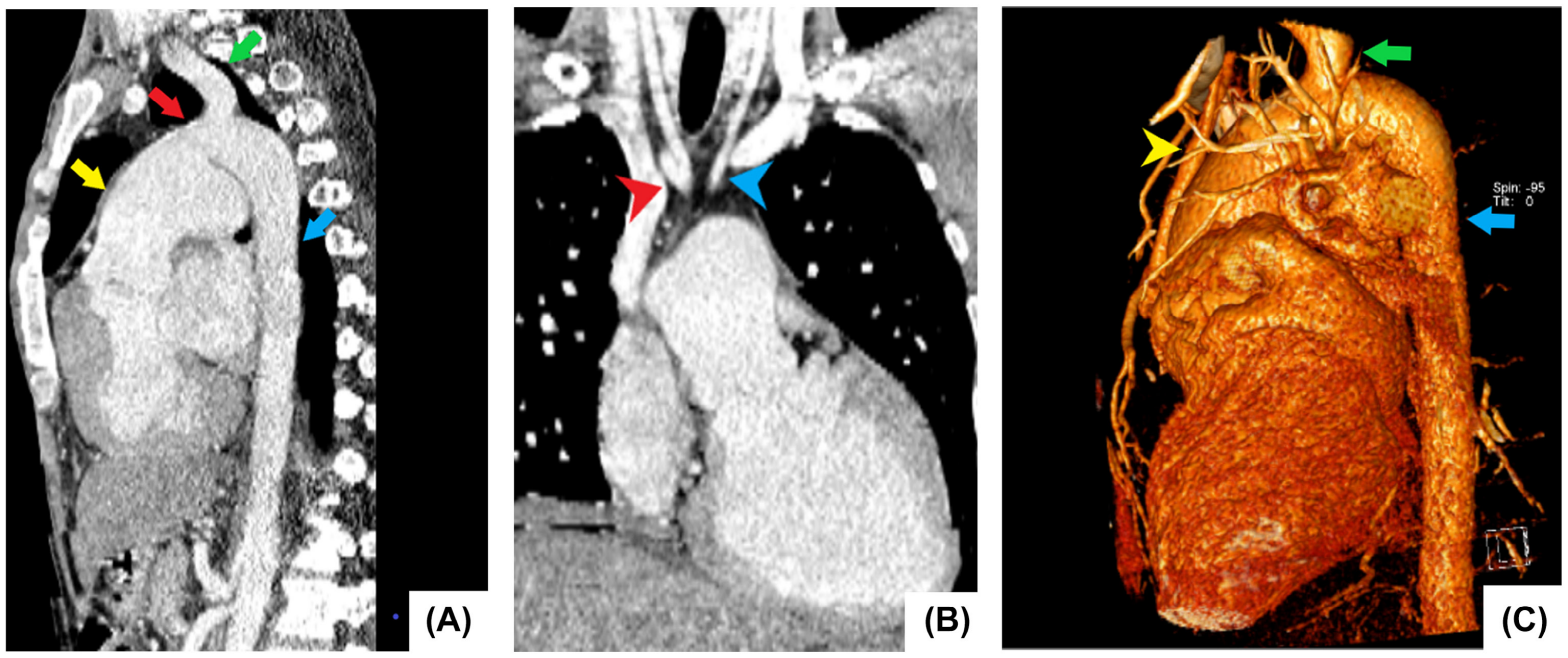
Cardiothoracic CT scan. Reconstructed images show interrupted aortic arch type B, (A) patent ductus arteriosus (red arrow) provides blood flow from the pulmonary artery (yellow arrow) to the left subclavian (green arrow) and descending thoracic arteries (blue arrow). (B) Brachiocephalic (red arrowhead) and left common carotid (blue arrowhead) arteries arise from the proximal aortic arch. (C) In the 3D image, there is an apparent interruption of the aortic arch between the hypoplastic ascending aorta (yellow arrowhead) and descending aorta (blue arrow) proximal to the left subclavian artery (green arrow).
